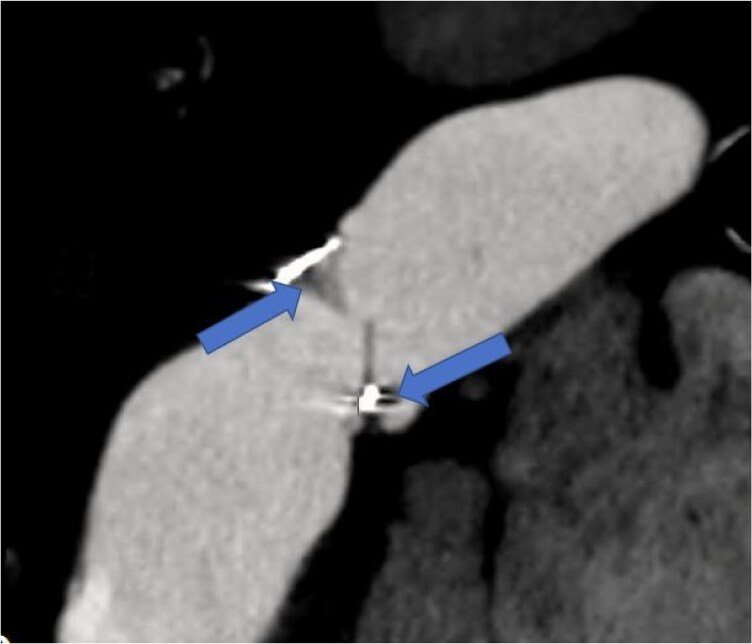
Cardiac computed tomography showing bioprosthetic pulmonic valve degeneration with pannus formation (arrows) on the leaflets resulting in significant mal-coaptation.
